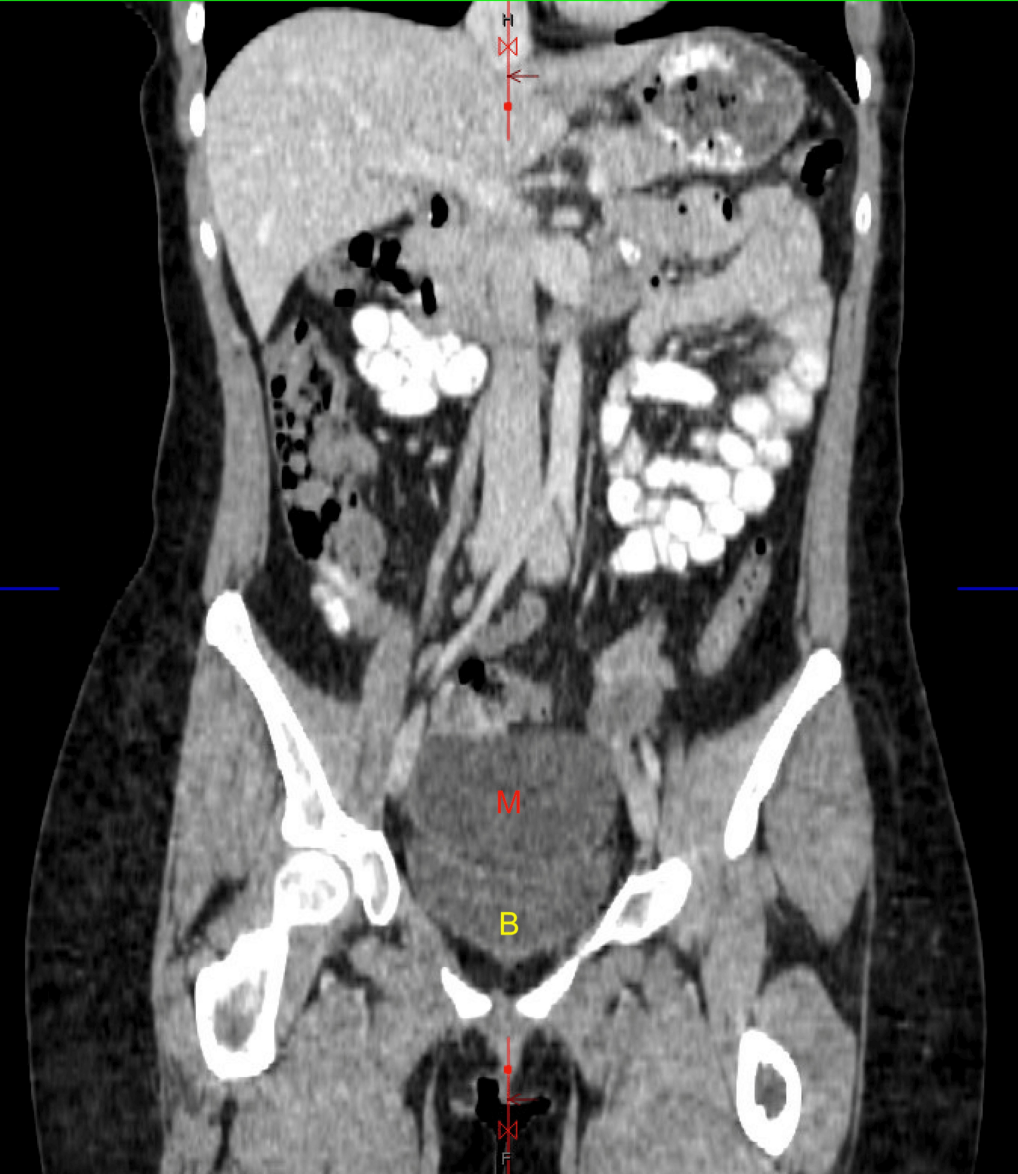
Coronal CT scan of the pelvis demonstrating a pelvic mass (M) exerting mass effect on the bladder (B)
